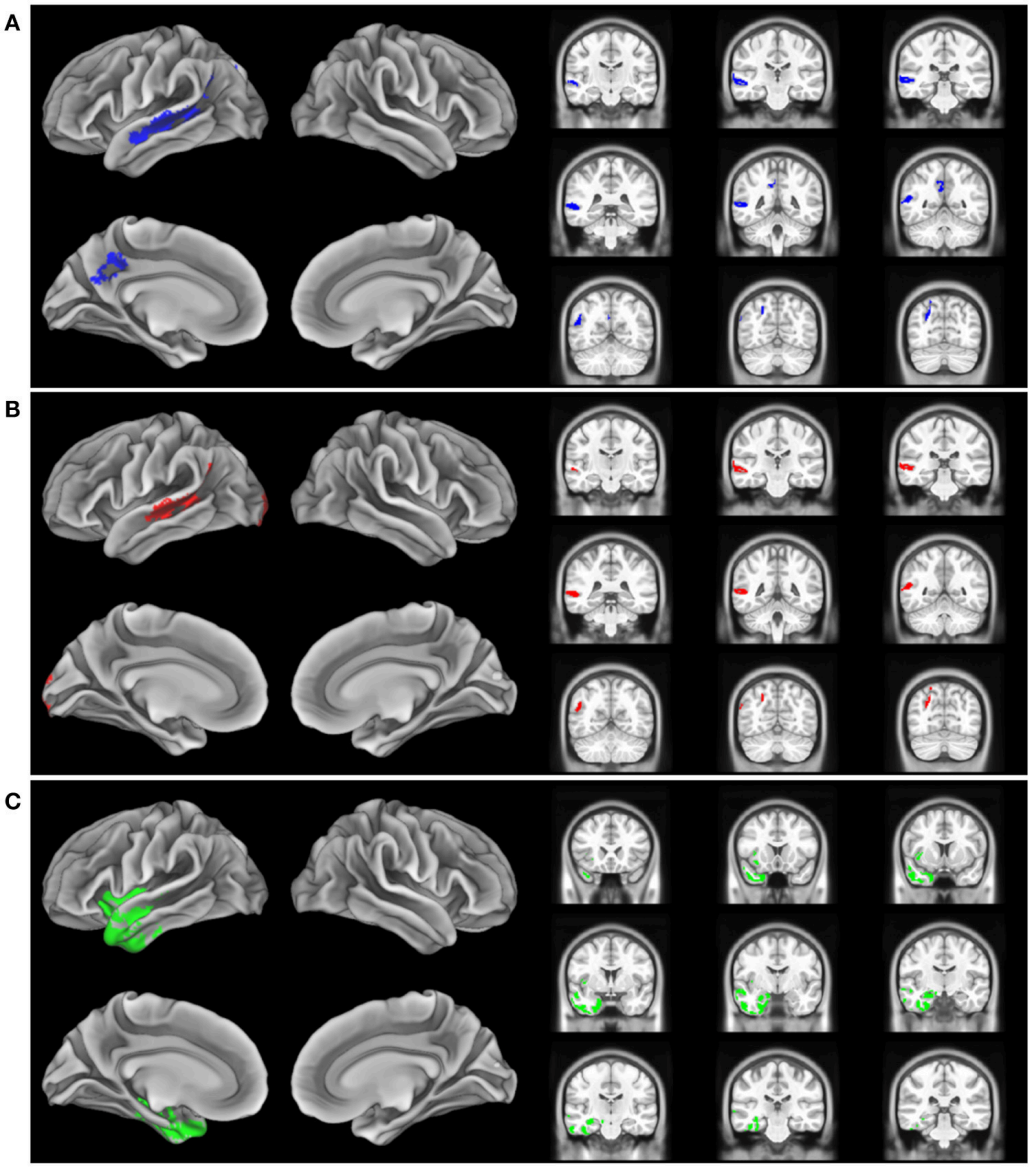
Distribution of significant gray matter atrophy in T1-weighted MRI scans. (A) low CSF Aß1-42 (<192 pg/mL) vs. controls, (B) logopenic variant PPA vs. controls, (C) High CSF Aß1-42 (>192 pg/mL) vs. controls.
